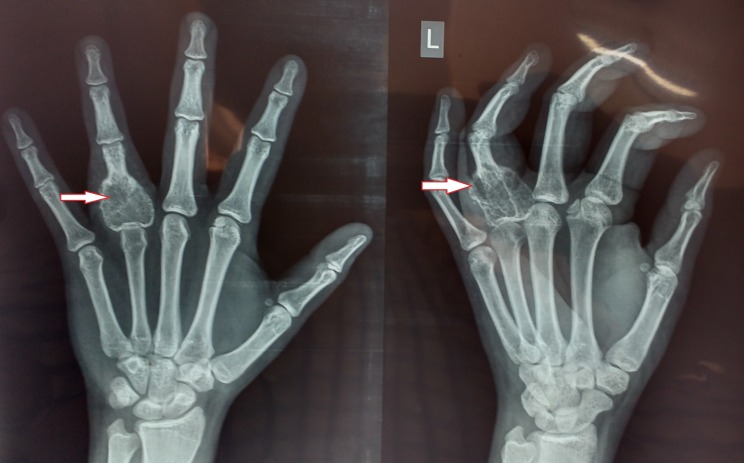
Pre-op radiograph (anteroposterior and oblique views) Shows lytic lesion at the base of the proximal phalanx of the ring finger
